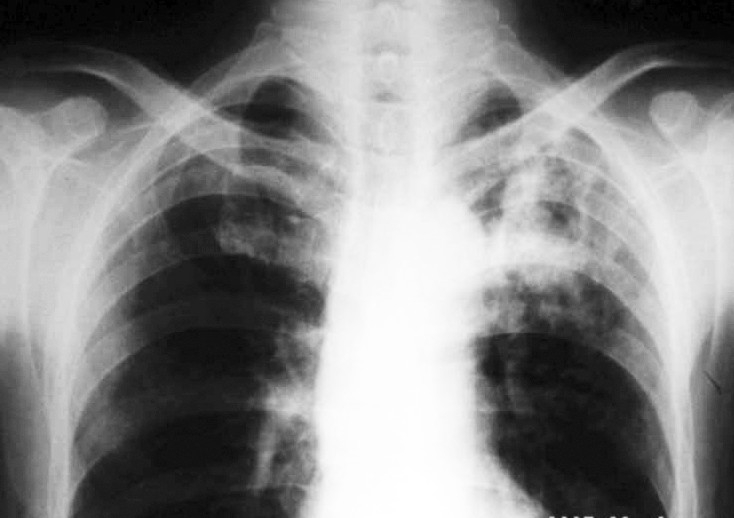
X-ray chest PA view showing non-homogenous opacity, left upper zone
